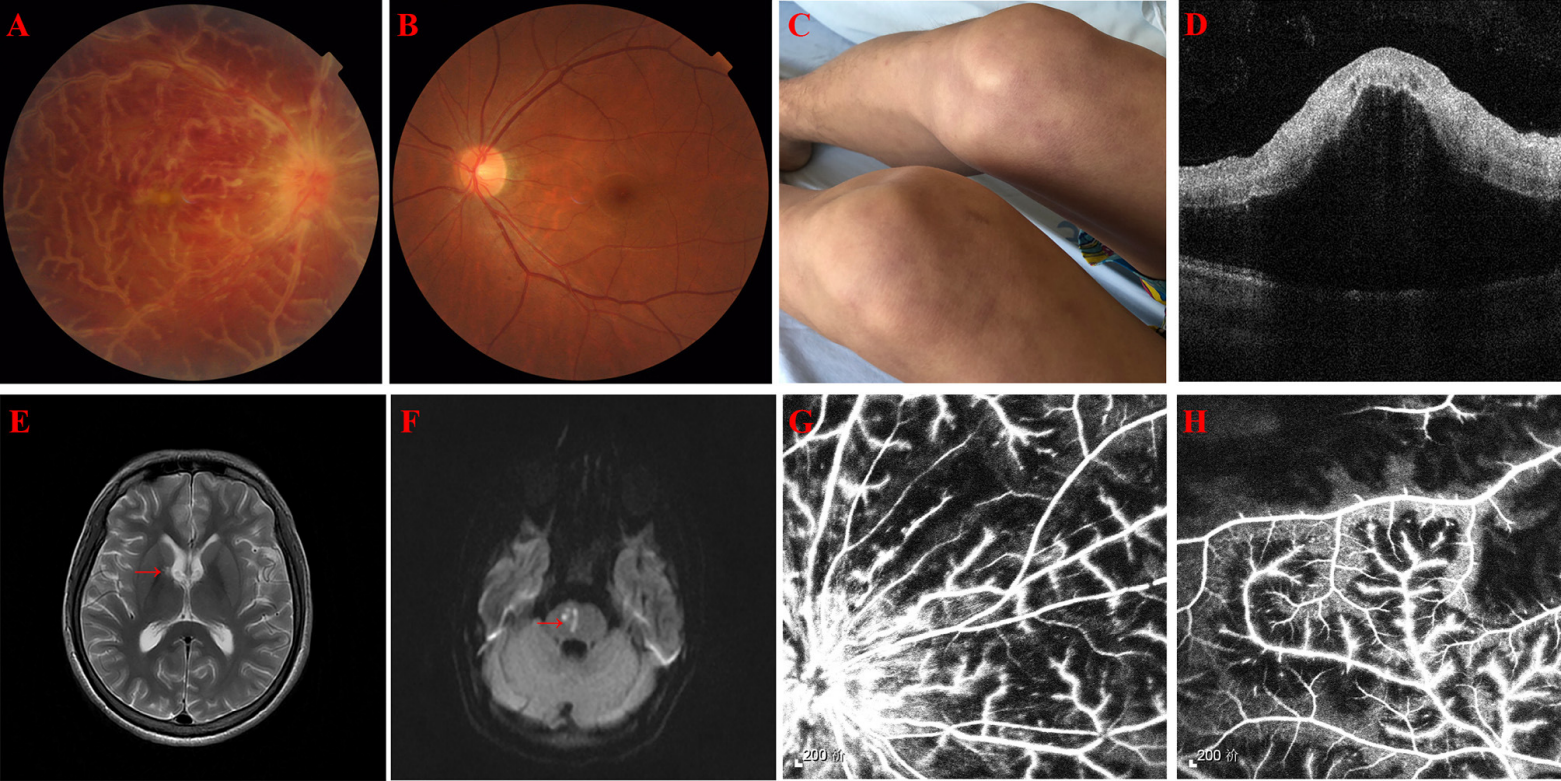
(A) A fundus photograph of the right eye showing thick, white confluent sheathing around retinal veins suggestive of frosted branch angiitis, disc hyperemia, and macular edema. (B) A fundus photograph of the left eye showing normal appearance. (C) Recurrent skin rash on both thighs. (D) Optical coherence tomography of the right eye showing exudative macular detachment, vitreous cells, and posterior vitreous detachment. (E) Old lacunar infarction involving the right basal ganglia and the lateral ventricle (red arrow) in magnetic resonance (MR) T2 phase, but not in the diffusion-weighted imaging (DWI) (image not showed). (F) Fresh lacunar infarction in the right pons (red arrow) in MR DWI. (G,H) Fluorescein angiography of the right eye showing extensive vascular leakage and supratemporal capillary non-perfusion areas.
